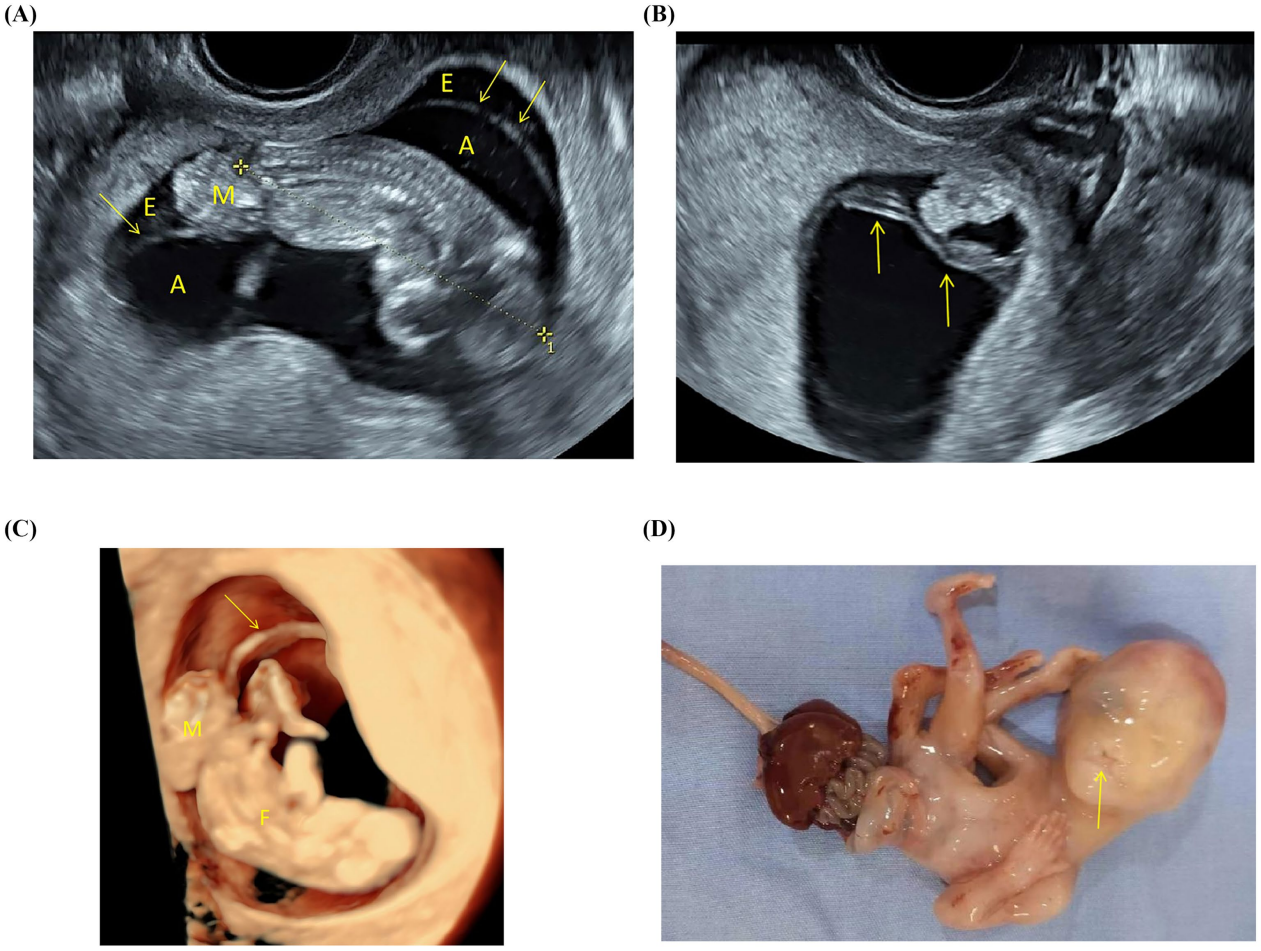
Routine fetal ultrasound examination of a 32-year-old woman in the first trimester. (A) Grayscale ultrasound shows that the fetal crown-rump length is shorter than expected for the gestational age in weeks. There is a large abdominal wall defect, with abdominal contents herniating into the unobliterated extraembryonic coelom (the arrows show the amniotic sac). A-amniotic cavity; E-extraembryonic coelom; M-mass. (B) The umbilical cord is short (arrows), lacks coiling, and is closely adhered to the amniotic membrane. (C) Three-dimensional ultrasound shows an abdominal extrusion of the fetus within the extraembryonic coelom (the arrow shows the amniotic sac), along with one lower limb displaying an abnormal posture. F-fetus; M-mass. (D) Gross examination follows induced labor demonstrates a fetal abdominal wall defect with visceral protrusion, limb malrotation, and a very short umbilical cord. Additionally, the fetus has a midline cleft lip and palate (arrow).
